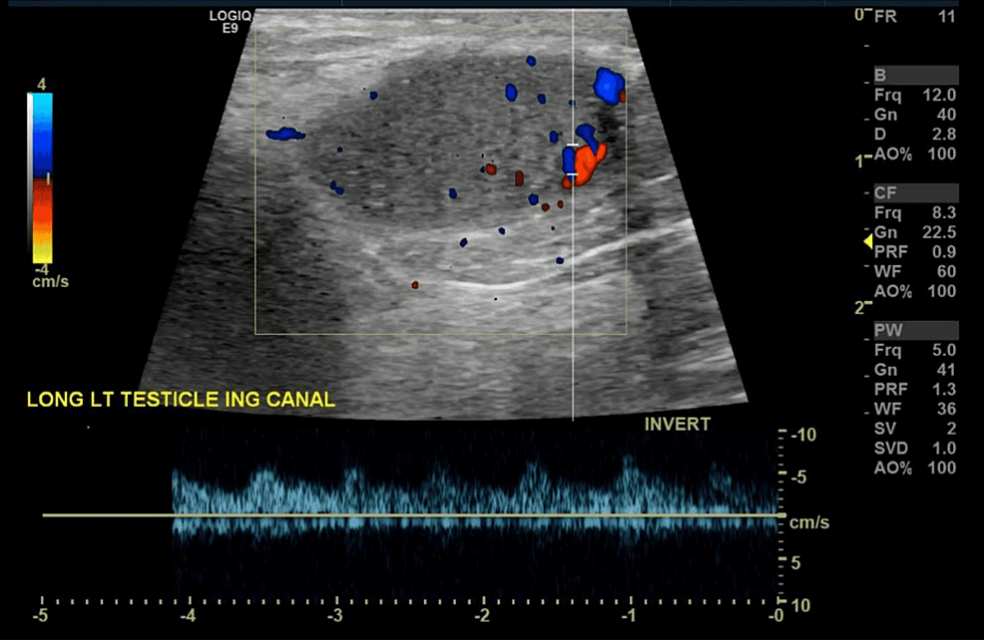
Color Doppler study image Scattered punctate hyperechoic foci and central color Doppler vascularity can be seen involving the inferior pole of the left testicle.
